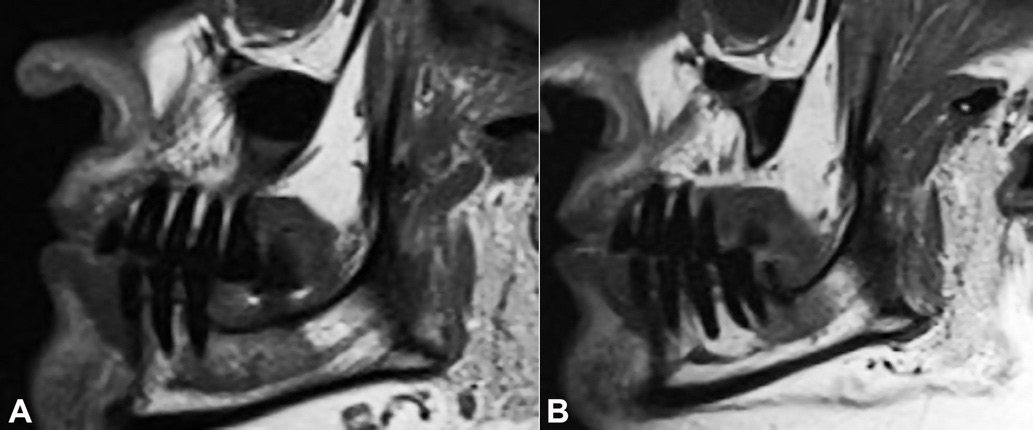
A – Left and B – right sagittal section of MRI (T1 weighed images). In both images, asymmetry of the mandibular canals is observed, with more evidence on the right side
